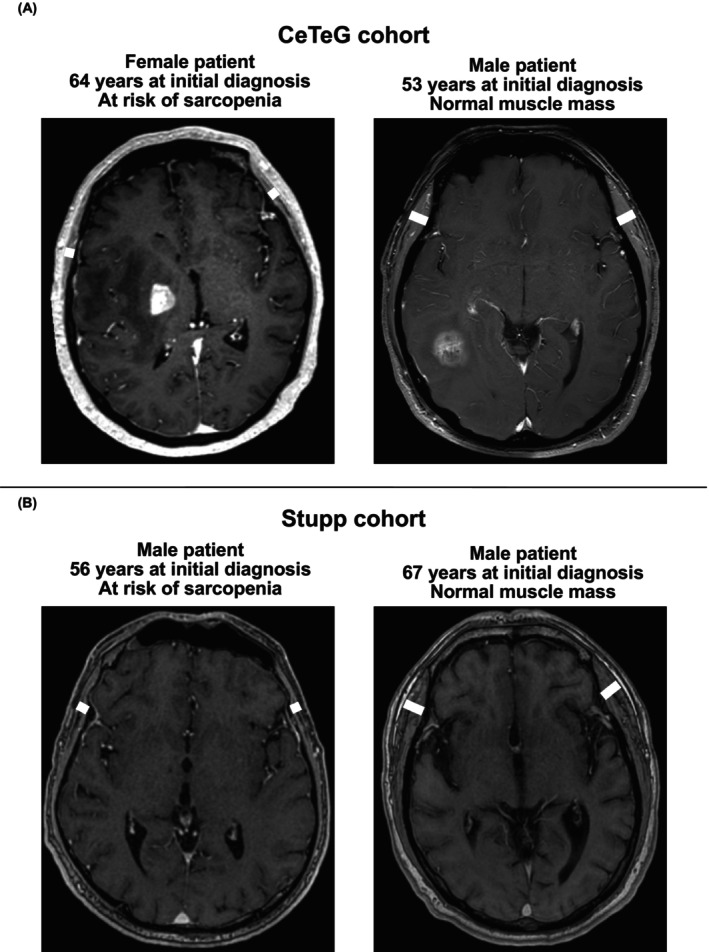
Examples of TMT assessment for patients from the CeTeG and Stupp cohorts. (A and B) indicate examples of patients at risk of sarcopenia (left) and patients with normal muscle mass (right) from the CeTeG (above) and Stupp cohort (below). TMT measurement was assessed on axial T1‐weighted contrast‐enhanced cranial MRI. The white lines indicate the exact calculation of TMT for both sides. MRI: Magnetic resonance imaging; TMT: Temporal muscle thickness.
